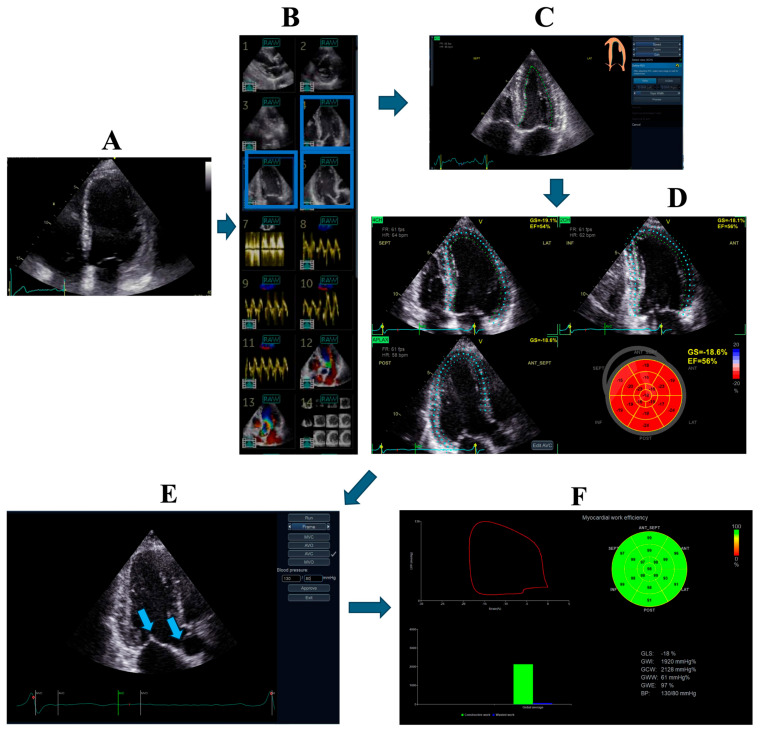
Example of automated calculation of left ventricular myocardial strain and myocardial work using the two-dimensional viewing in echocardiography. (A) Manual selection of a four-chamber view by the operator. (B) Automatic retrieval of the additional optimal apical views (two- and three-chamber) from the stored dataset (matching cardiac cycle and frame rate requirements, highlighted in blue). Panels 1–6 show 2D echocardiographic views; 7–11 spectral and tissue Doppler; 12–13 color Doppler; and 14 serial short-axis views derived from the apical long-axis. (C) Automated endocardial and epicardial border delineation in systole and diastole across all views. (D) Automated quantification of global and segmental strain with graphical dis-play. At steps C and D, the operator can edit and correct the measurements if needed. (E) Input of brachial arterial blood pressure and automated or manual timing of aortic and mitral valve open-ing/closure. Arrows indicate aortic and mitral valves. (F) Display of strain-derived pressure–volume loops and myocardial work indices (global constructive work, wasted work, global work efficiency).
